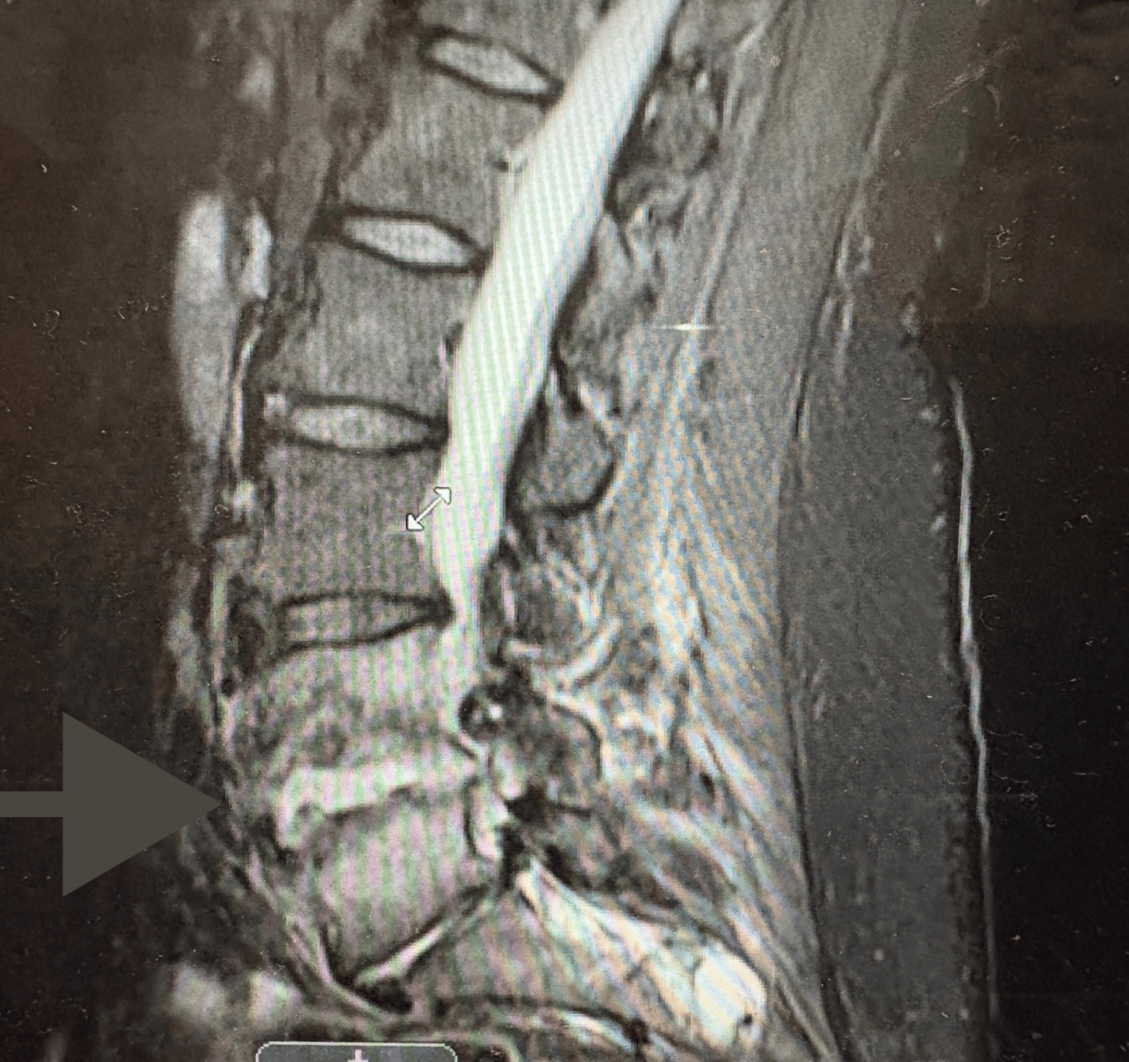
STIR sequence MRI of the lumbar spine at L4-L5 demonstrating vertebral discitis and osteomyelitis, confirming bilateral psoas abscesses with rim enhancement and inflammatory changes extending into the paraspinal musculature STIR, short tau inversion recovery
